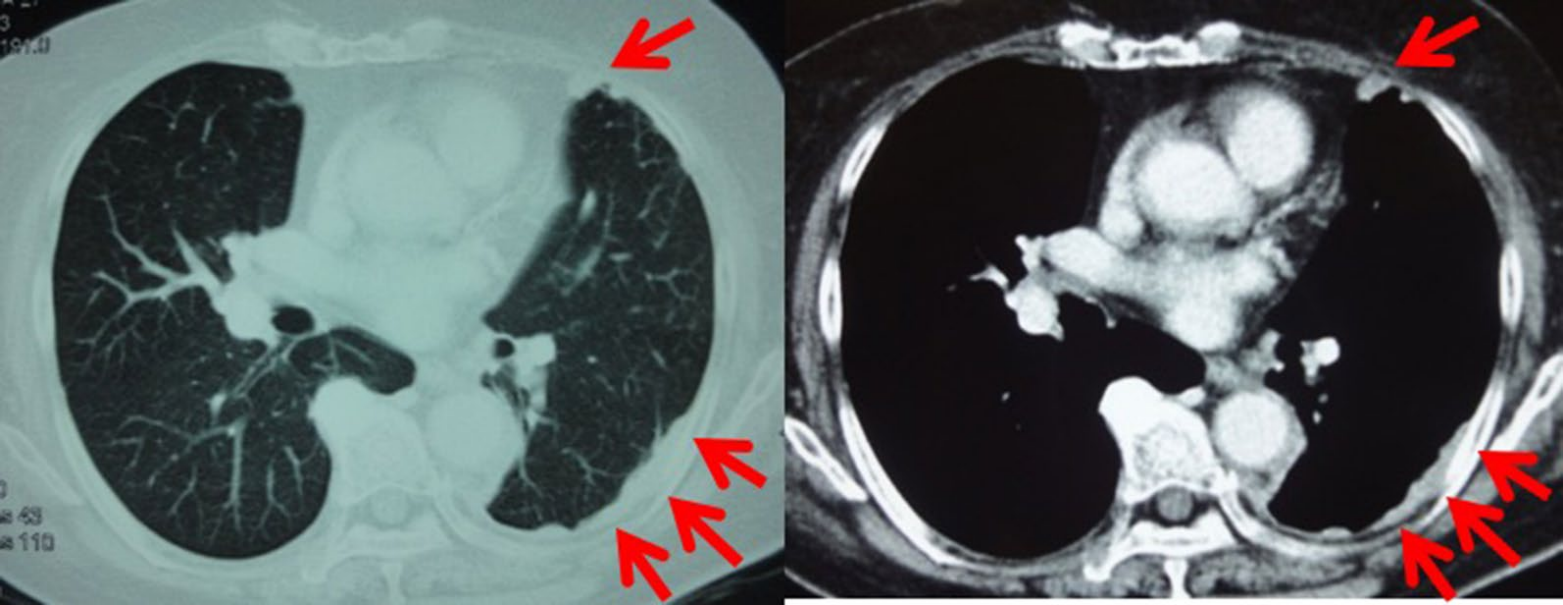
Pleural metastasis was observed in the postoperative chest CT scan. Arrows (→) show pleural metastasis and pleural effusion
